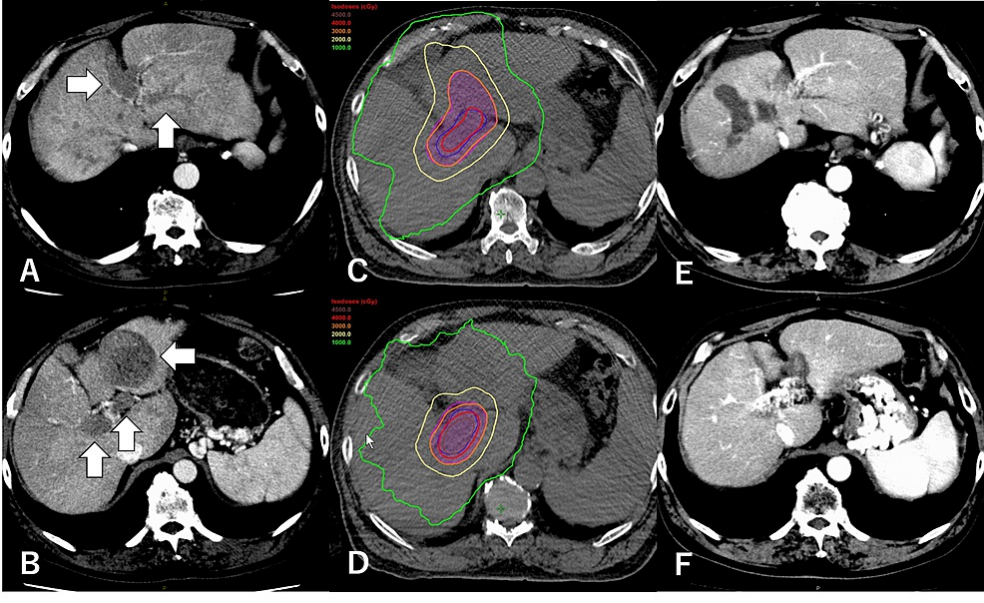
Case 3 – hypofractionated radiotherapy followed by multidisciplinary treatments A, B: pretreatment CT of the liver demonstrating hepatocellular carcinoma with massive portal vein tumor thrombosis from the main trunk to the secondary branches of both lobes (arrows). C, D: axial images of the dose distribution of radiotherapy. Hypofractionated radiotherapy was performed with 30 Gy in 10 fractions prescribed to enclose the planning target volume with a 60% isodose line of the maximal dose equated to the prescribed dose. E, F: the latest CT scan with no recurrence after 48 months after radiotherapy. Portal vein invasion has been thrombosed and occluded, with collateral blood vessels being formed CT: computed tomography
